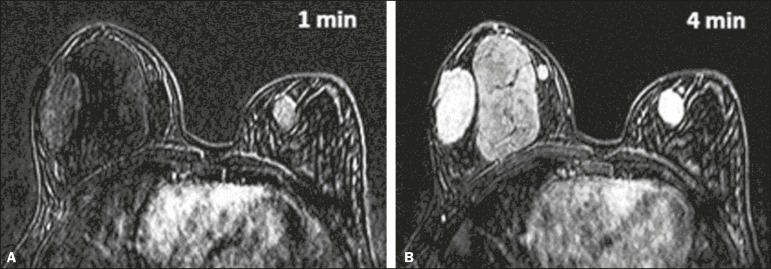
Magnetic resonance imaging identifying lesions with the same morphology
